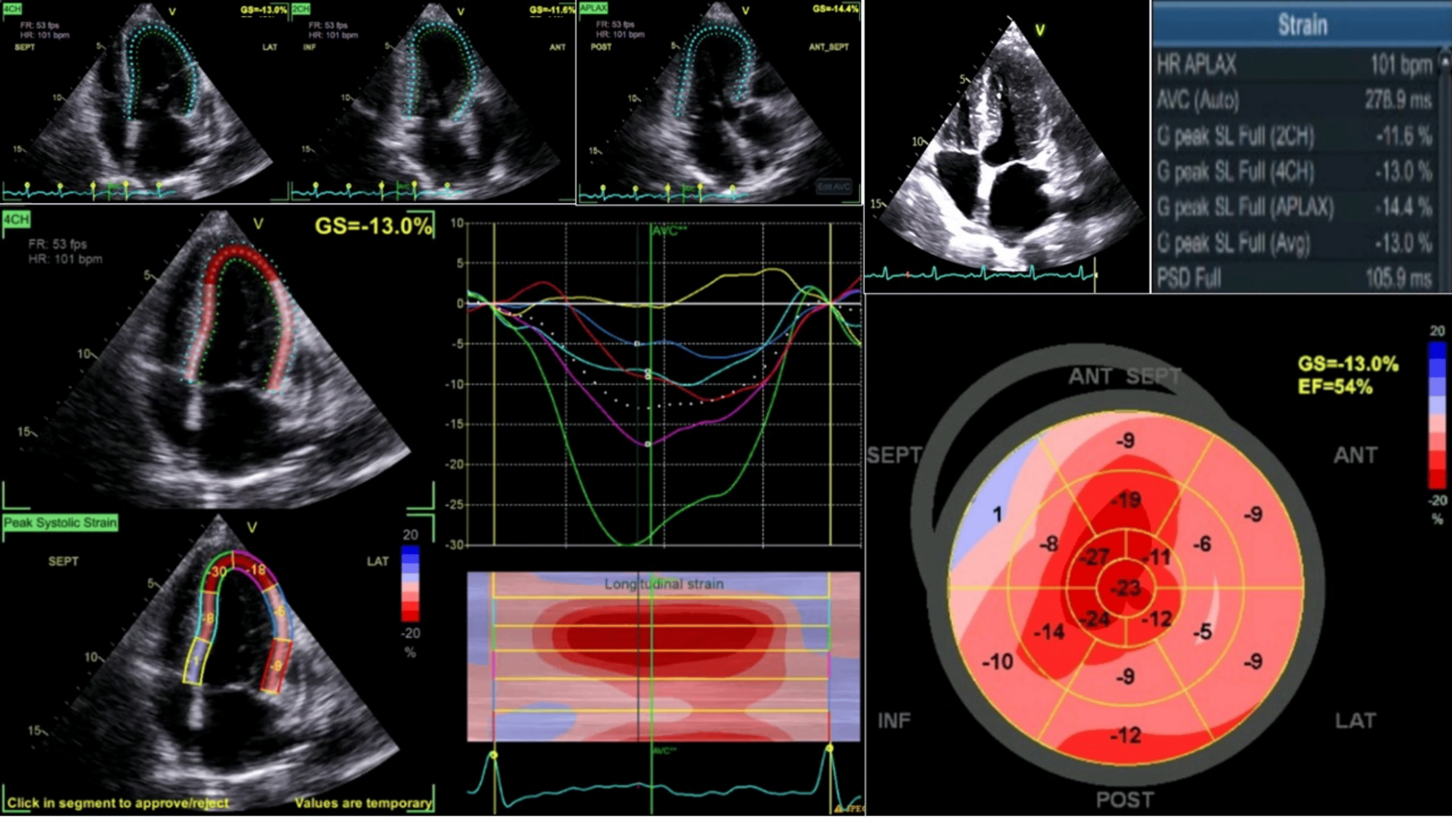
Transthoracic echocardiography revealed concentric left ventricular hypertrophy with preserved systolic function and a characteristic “apical sparing” pattern of global longitudinal strain, consistent with cardiac amyloidosis. HR, heart rate; PSD, peak strain dispersion; GS, global strain; EF, ejection fraction; ANT, anterior segment; INF, inferior segment; LAT, lateral segment; POST, posterior segment; SEPT, septal segment; G peak SL, global peak strain longitudinal; AVC, aortic valve closure; HR APLAX, heart rate at apical long-axis view; LVP, left ventricular pressure
